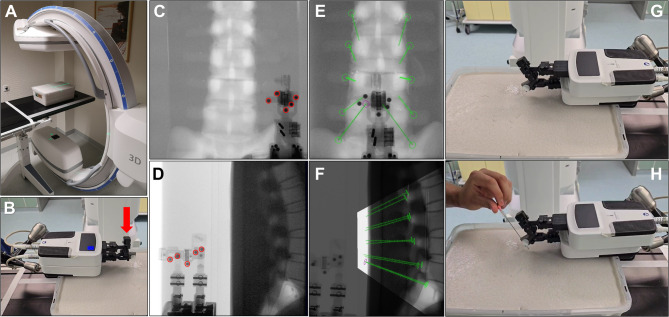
(A) Experimental setup with the lumbar spine model placed on a carbon table and the C-arm in AP position. (B) Interventional robot mounted above the model using a support arm. Red arrow = movable robotic end-effector. (C) AP X-ray image showing the robot’s registration markers. (D) Lateral X-ray image showing the robot’s registration markers. (E) Planning of needle trajectories in the robot software, AP view. (F) Planning of needle trajectories, lateral view. (G) Robot aligning itself to the planned trajectory. Note the tilted end-effector. (H) Insertion of the puncture needle into the end-effector’s guide.
